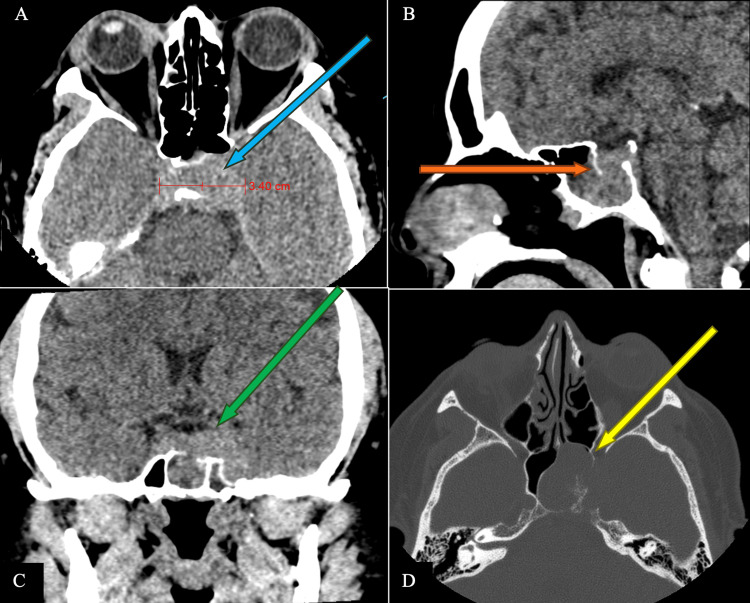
(A) Axial CT of the brain and paranasal sinuses shows a mildly hyperattenuating, soft tissue, expansile mass arising from the sella turcica (blue arrow). (B) Sagittal CT image demonstrates expansion through the floor of the sella turcica (orange arrow) with extension into the left sphenoid sinus inferiorly. (C) Coronal CT image shows lateral extension into the left middle cranial fossa (green arrow). (D) Axial bone window CT image shows expansile remodelling of the sphenoid bone (yellow arrow). CT: computed tomography
